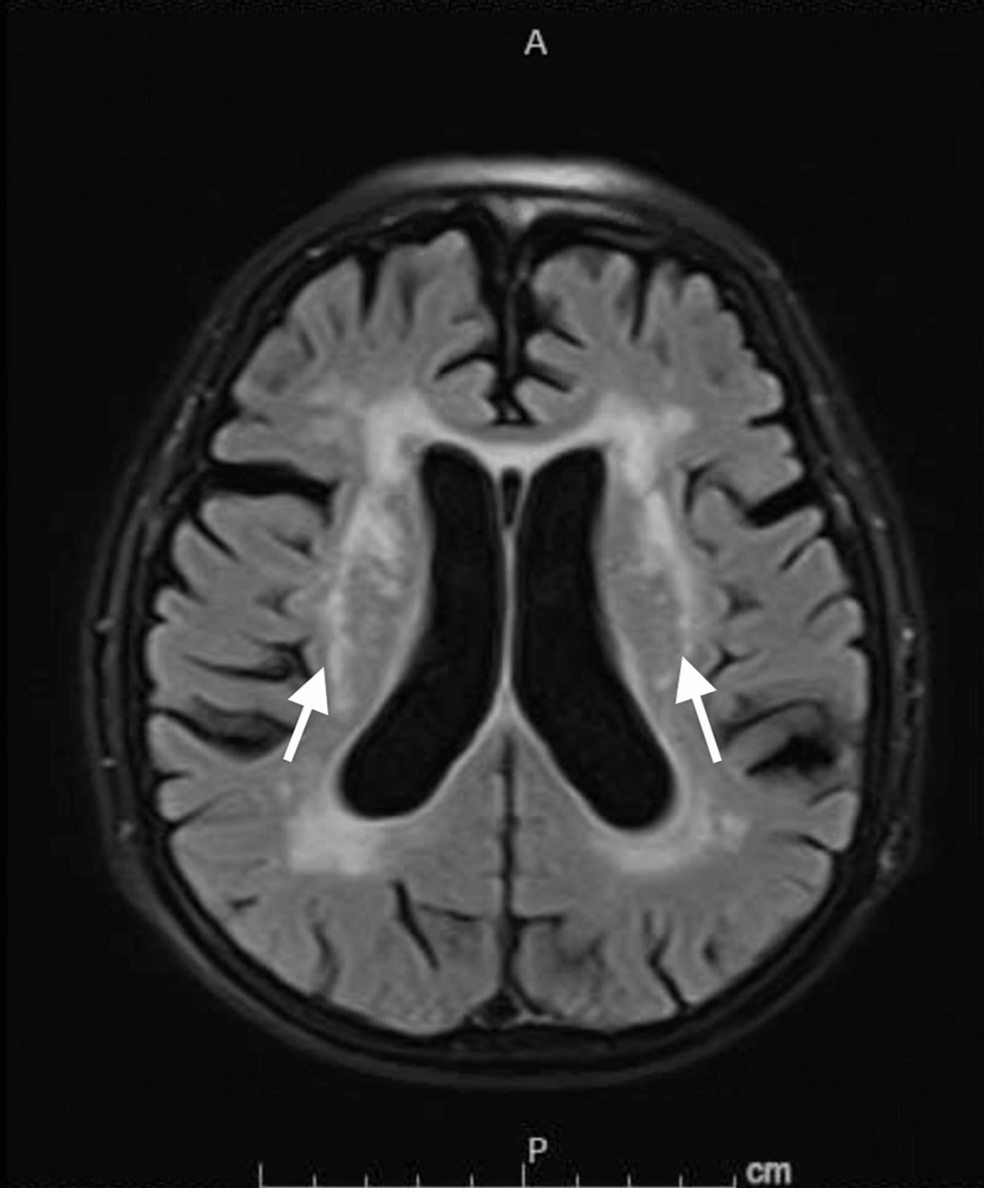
Magnetic Resonance Imaging of the Brain Magnetic resonance imaging (MRI) brain showing only bilateral periventricular old lacunae infarctions (arrows) with no other identifiable pathology explaining the clinical picture.
